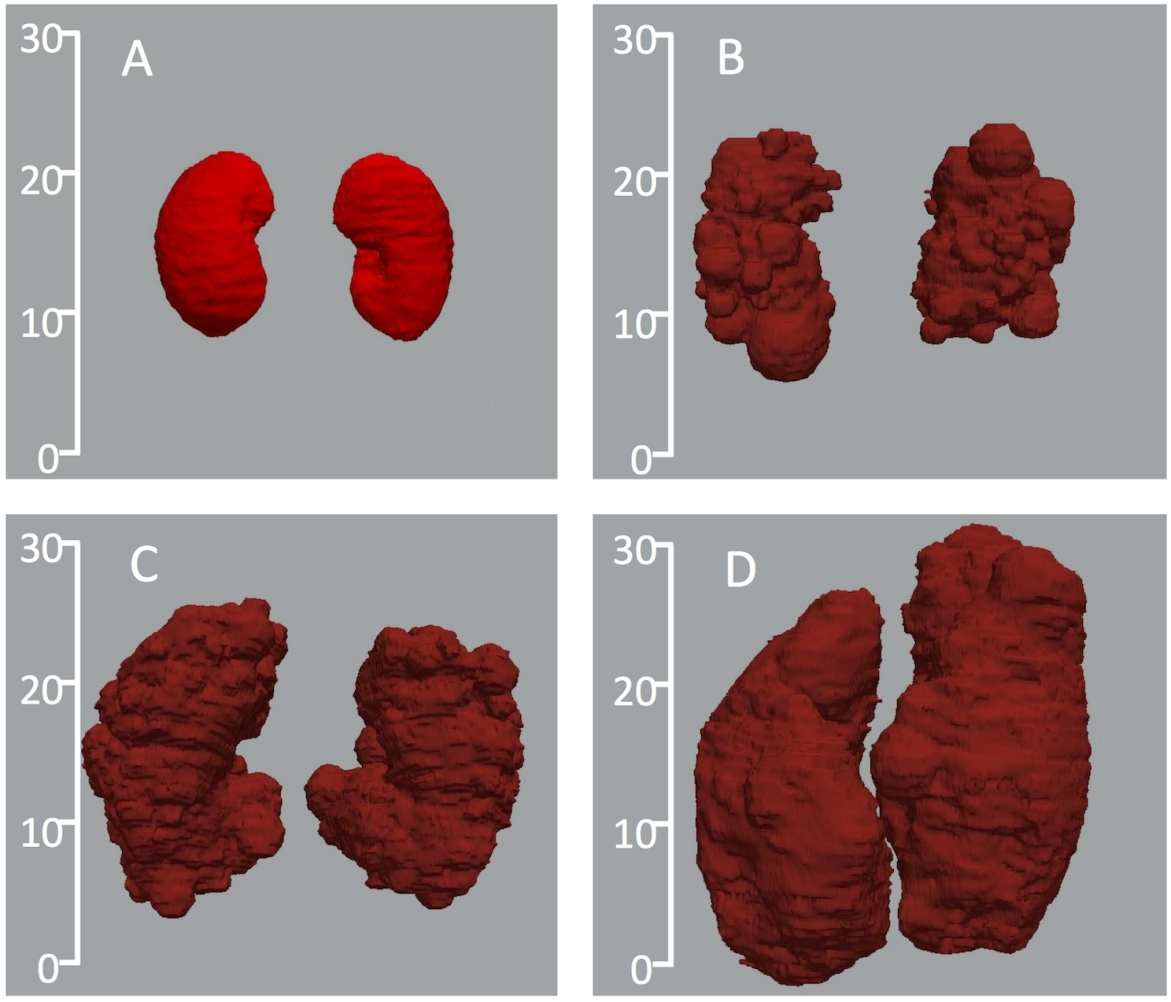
Three-dimensional representation of ADPKD kidneys in comparison with normal kidneys. Scales represent dimension in cm. The kidney shape, size, and volume highly differ between the normal control (panel A: total kidney volume (TKV) = 591 ml) and the patients (panel B: TKV = 1327 ml; panel C: TKV = 3026 ml; panel D: TKV = 5836 ml). Kidneys were reconstructed using VMTK software based on binary masks obtained from ImageJ polyline manual tracings on representative CT images.
